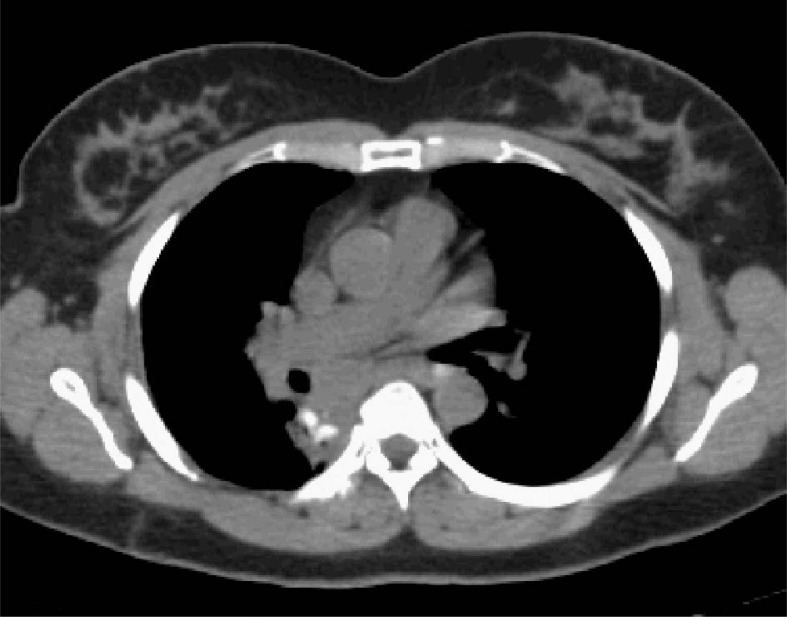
CT at four months post-RT. The mass is noted to be smaller.
